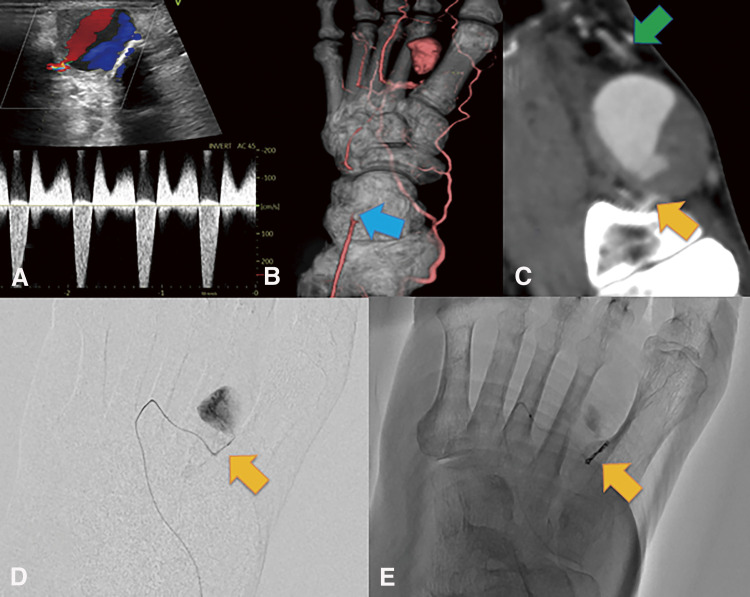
Pre- and intra-embolization findings. (A) Echogram indicating a 29 × 18 × 20 mm saccular aneurysm with a to-and-fro blood-flow waveform. (B) Contrast-enhanced computed tomography reveals an aneurysm of the first dorsal metatarsal artery and an occluded dorsalis pedis artery (blue arrow). (C) The aneurysm is located in the middle segment of the first dorsal metatarsal artery. The orange and green arrows represent the proximal and distal dorsal metatarsal arteries, respectively. (D) Digital subtraction angiography of the aneurysm indicates no arterial drainage. The orange arrow indicates the first proximal dorsal metatarsal artery. (E) Embolization is performed for the proximal first dorsal metatarsal artery (orange arrow) with Target Nano 2 mm × 3 cm and 1.5 mm × 3 cm (Stryker; Boston, MA, USA).
